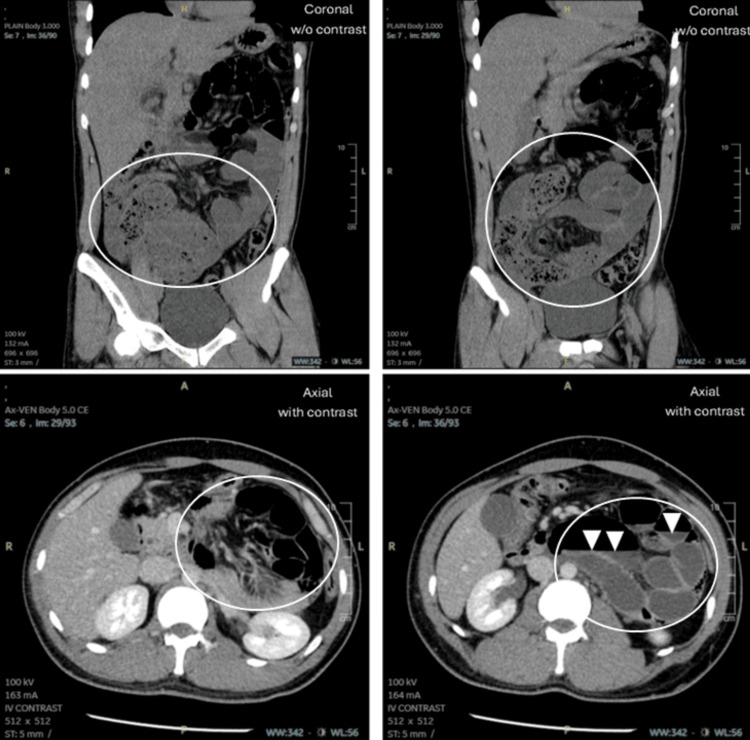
CT scan of the abdomen (coronal and axial views).
